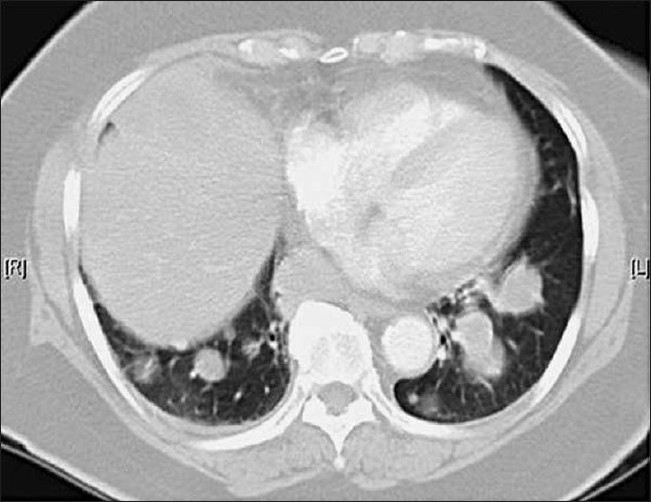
Computed tomography scan of chest
